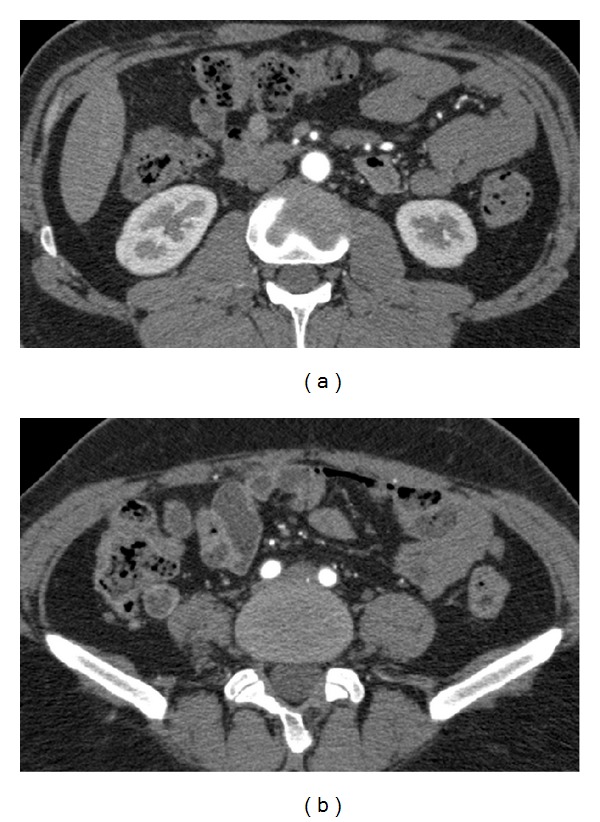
Axial CT angiographic sections show almost normal thickness of the wall of the aorta (a) and both common iliac arteries (b).
